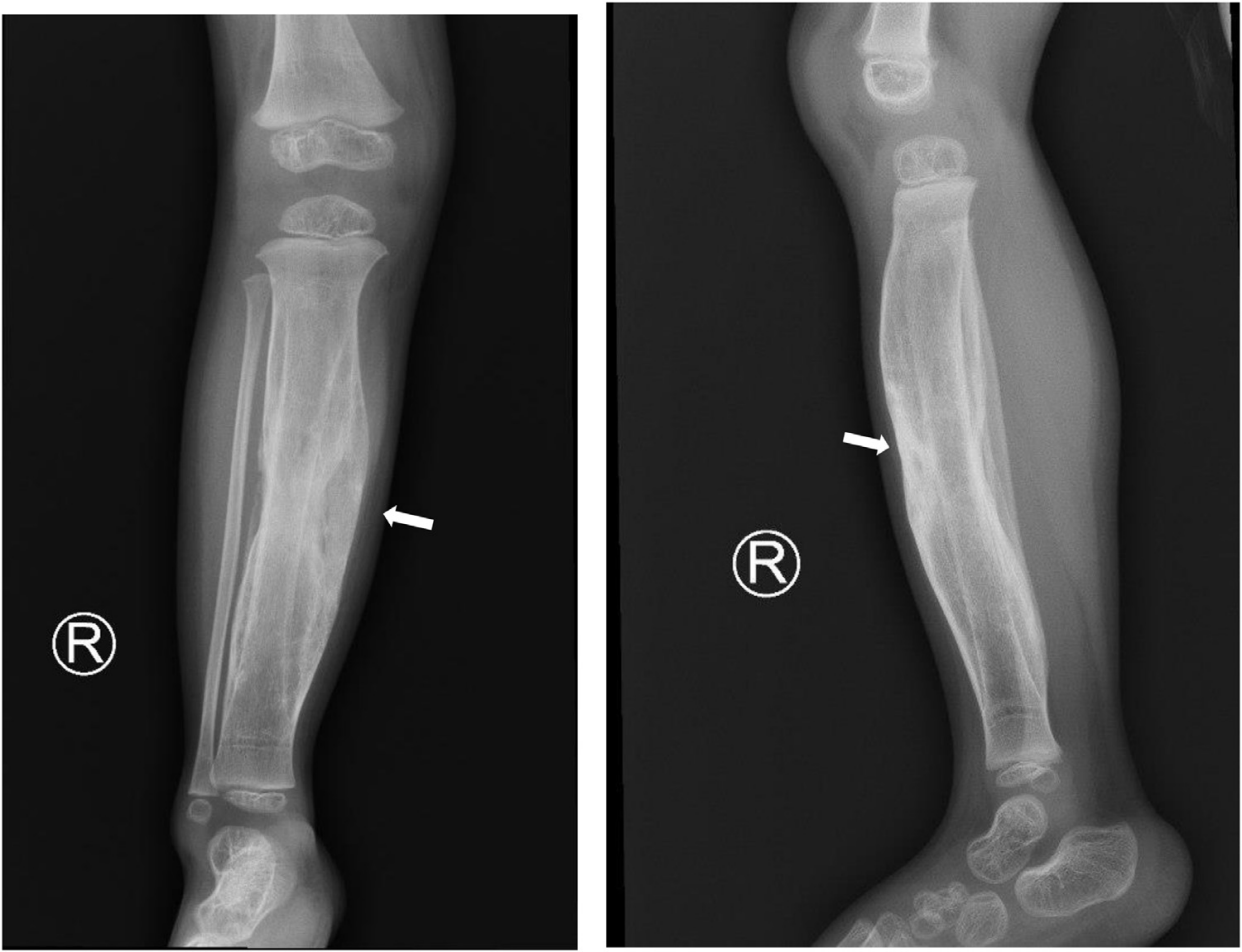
X-ray of the patient shows a soft tissue mass in the right cruris with peripheral calcification (arrow) causing compression and separation between the tibia and fibula.
